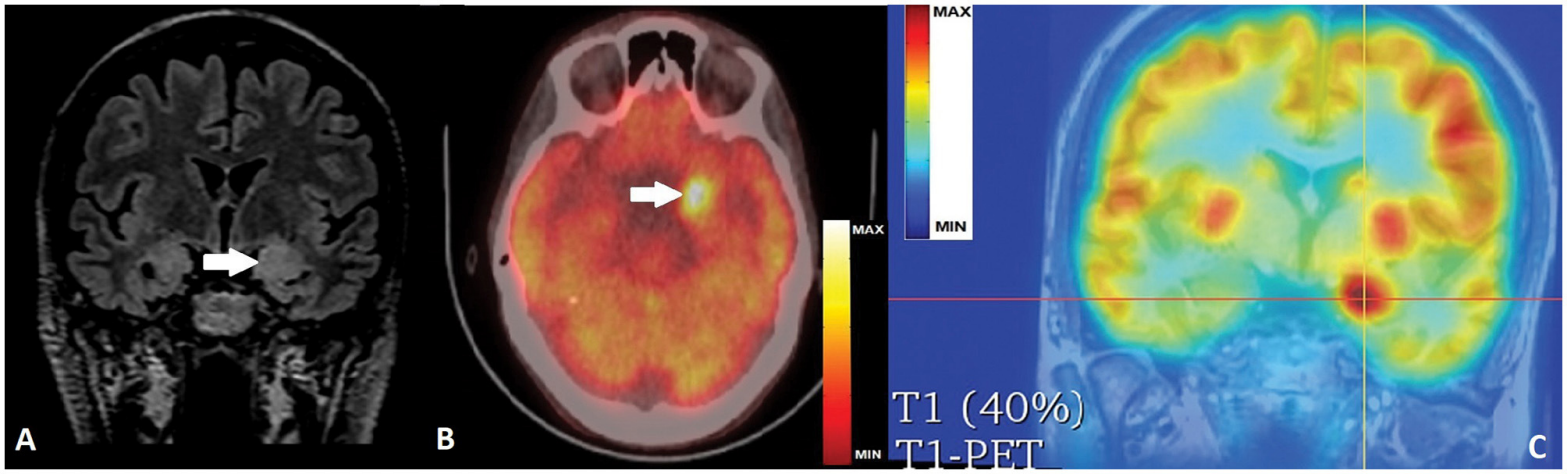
(A) Coronal FLAIR MRI with bi-mesiotemporal hyperintensity, more prominent on the left, with increased volume of left amygdala (white arrow). (B) Axial PET-CT scan with hypermetabolic left mesiotemporal spot(white arrow). (C) PET-T1 MRI co-registration with clear hyperactivity in the left amygdala (crossed lines' center).
